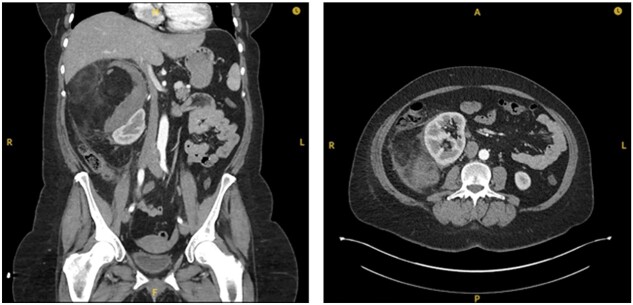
Interval CT imaging showing decrease in size of lesion and surrounding haematoma.
